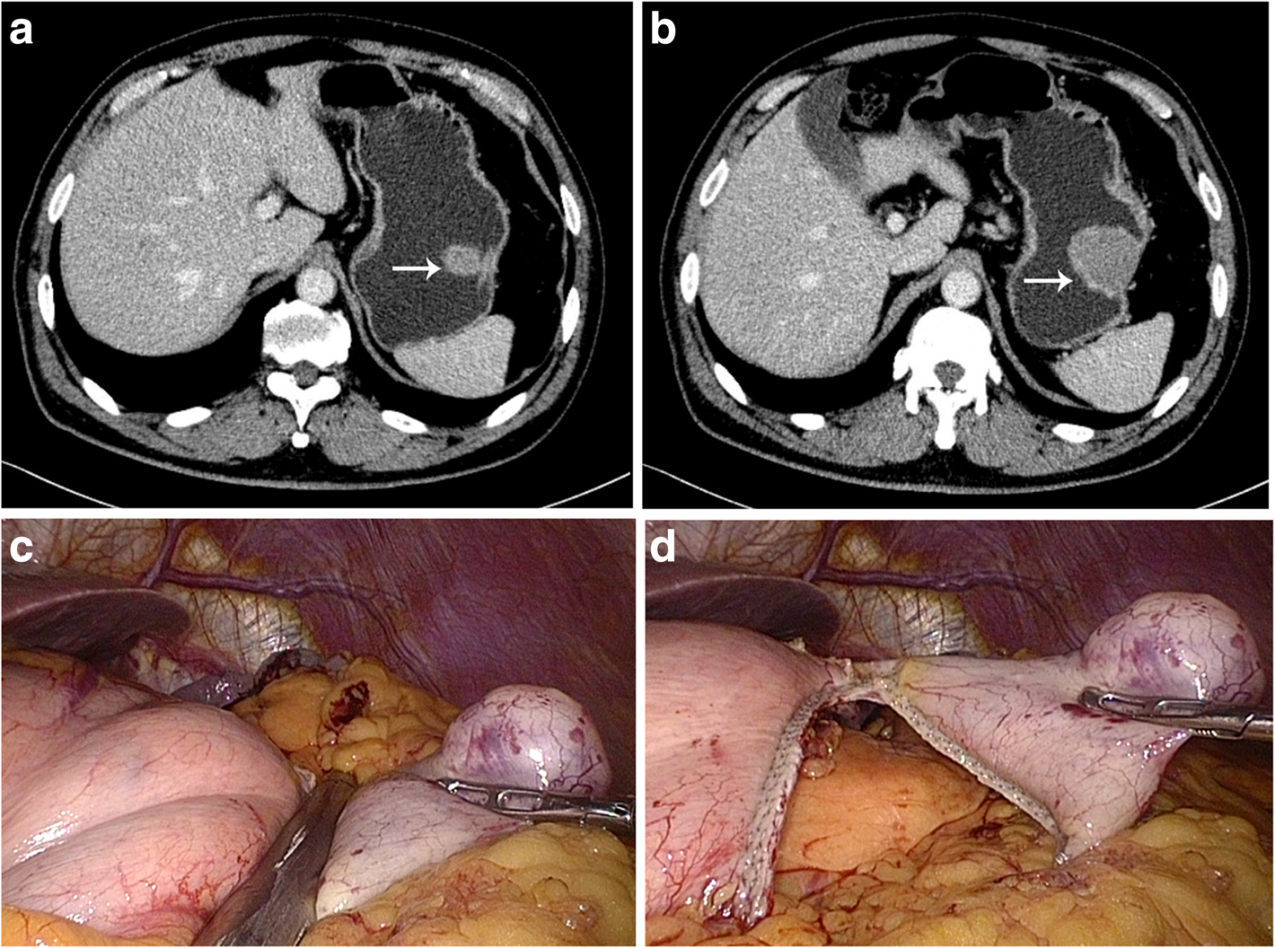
Resection of tumor in anterior wall of gastric body. (a) Image of the tumor from abdominal CT scan. (*white arrow*). (b) Image of the tumor from abdominal CT scan. (*white arrow*). (c) Resect the wall included the gastric SMTs using linear stapler. (d) Fire the anastomat and complete the resection
